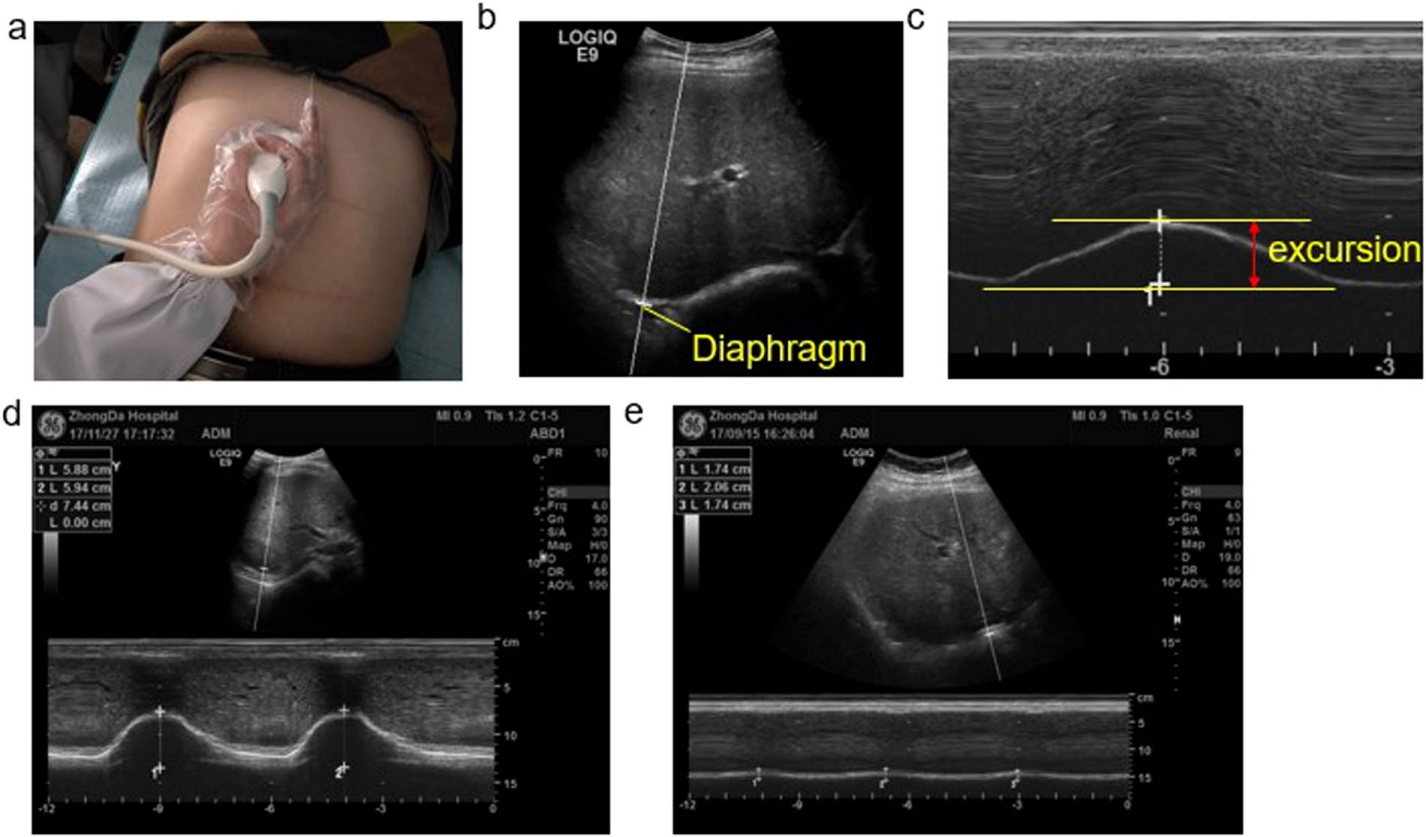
Diaphragm excursion measured by ultrasound decreases in HD patients during deep breathing. (a) The appropriate probe position for B and M mode diaphragmatic mobility measurements using a 1–5 MHz probe. (b) B-mode diaphragm sonography; the bright line reflects the diaphragm using the anterior subcostal approach. (c) M-mode tracing showing the amplitude of excursion during deep breathing. The arrows indicate the beginning and the end of diaphragmatic contraction, and the distance between the arrows indicates the diaphragm excursion. (d) Diaphragm excursion in a normal person during deep breathing. (e) Diaphragm excursion in a haemodialysis dialysis patient during deep breathing.
